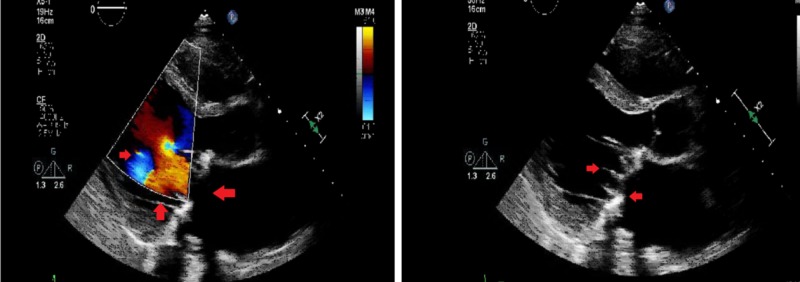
Transthoracic echocardiogram after valve replacement showing normal gradients across the replaced mitral valve that normally opens in diastole as well as dilated left atrium (left) and left ventricle (right)
